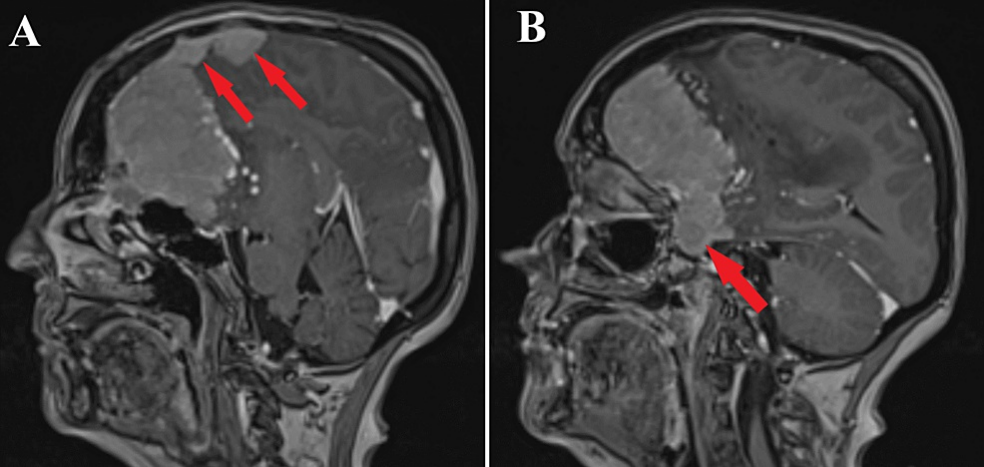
Preoperative MRI T1 post contrast sequence, sagittal section. A: Tumoral extension into the falx cerebri is represented (red arrows).
